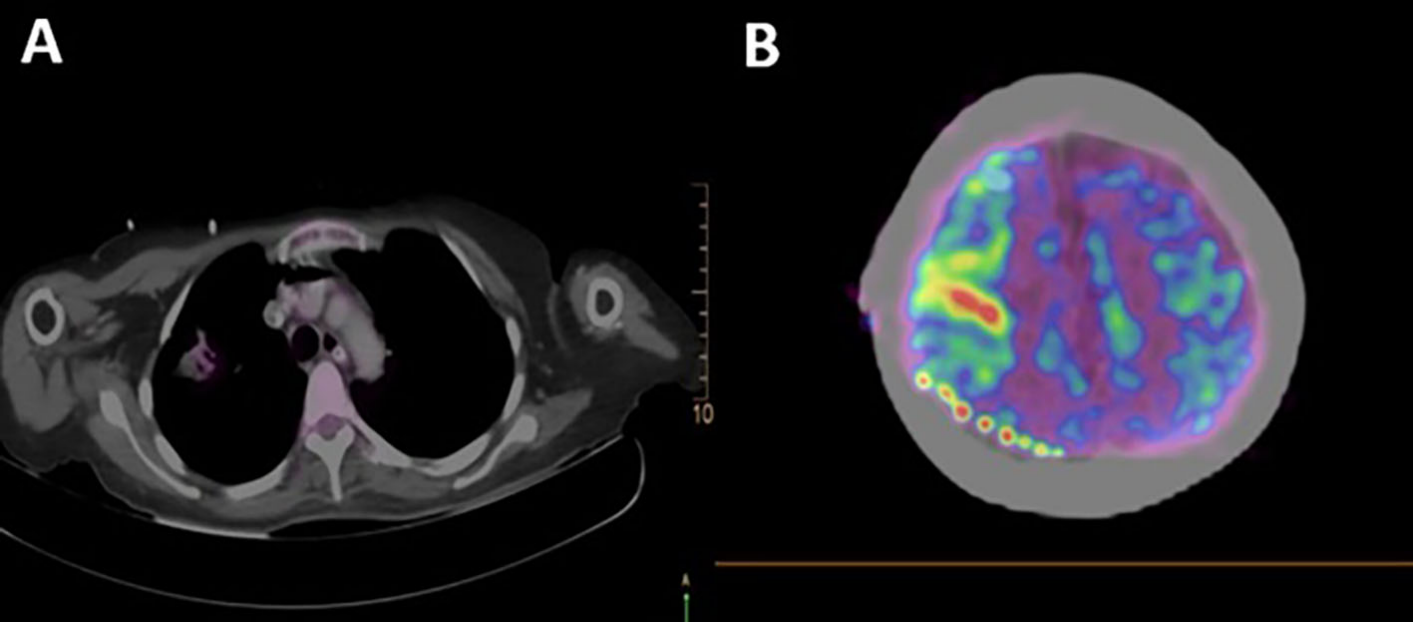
Total body 18F-fluorodeoxyglucose positron emission tomography (18F-FDG PET-CT): A. Pulmonary hypermetabolism without neoplastic mass in tomographic scanning (lung); B. FDG hypermetabolism at the right parietal region (cerebral).
